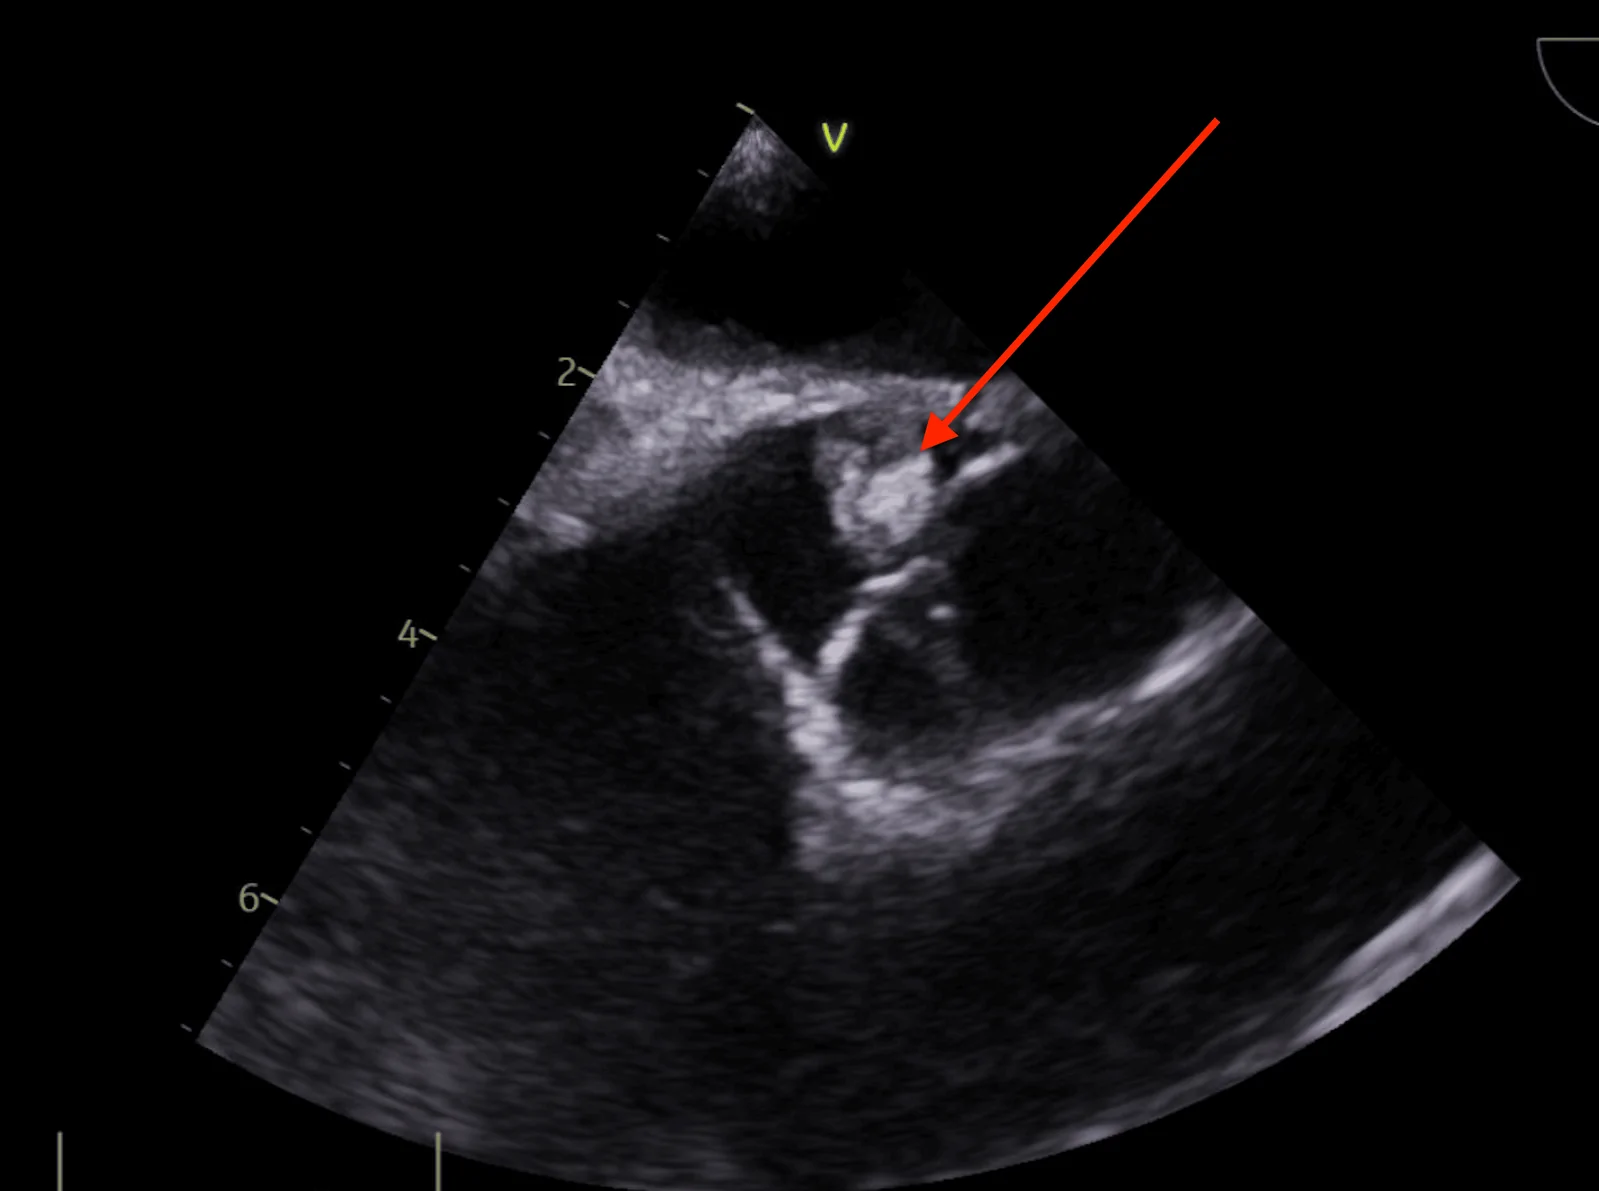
Transoesophageal echocardiography demonstrating native aortic valve infective endocarditis Mid-oesophageal views showing a mobile vegetation attached to the non-coronary cusp of the trileaflet aortic valve (arrow), with associated mild aortic regurgitation, confirming the diagnosis of aortic valve infective endocarditis.
